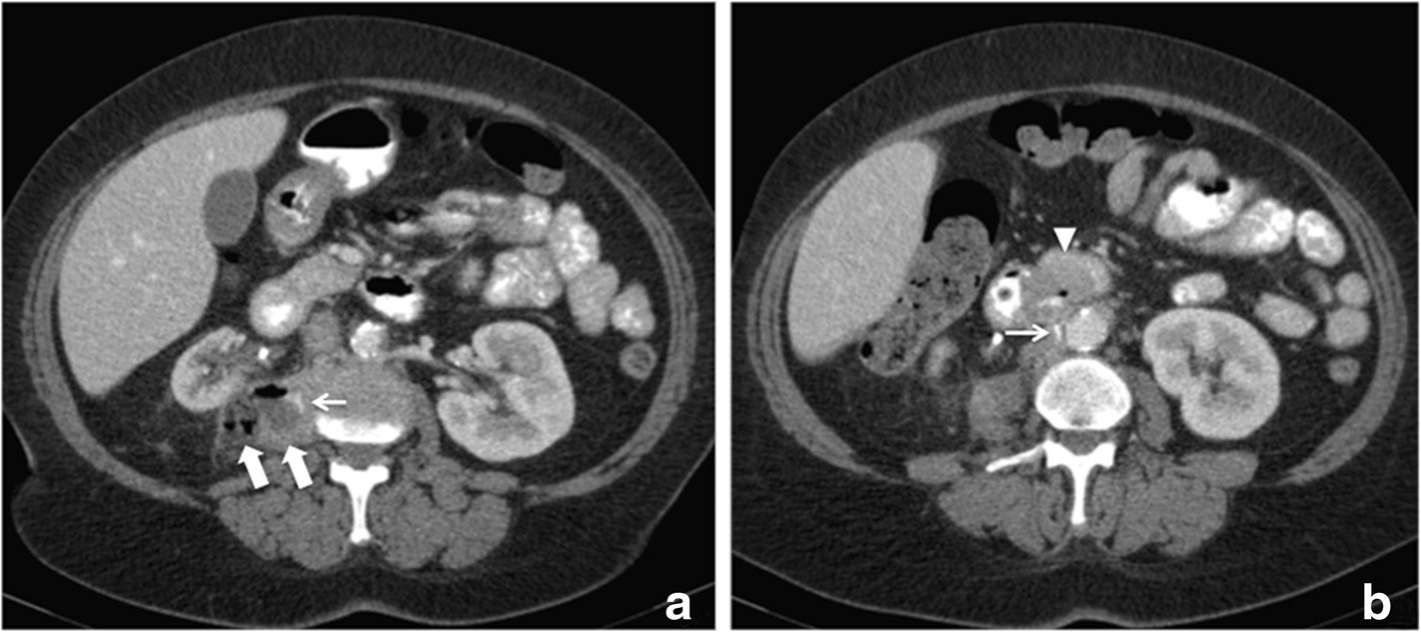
Abdominal CT scan at admission. Axial slices of a contrast enhanced computed tomography showing a 29 x 41 mm abscess (thick white arrow) in the right psoas muscle (a). Positive oral contrast media marking the fistula (small white arrow) from the thickened wall of the duodenum (white arrowhead) to the abscess (b)
